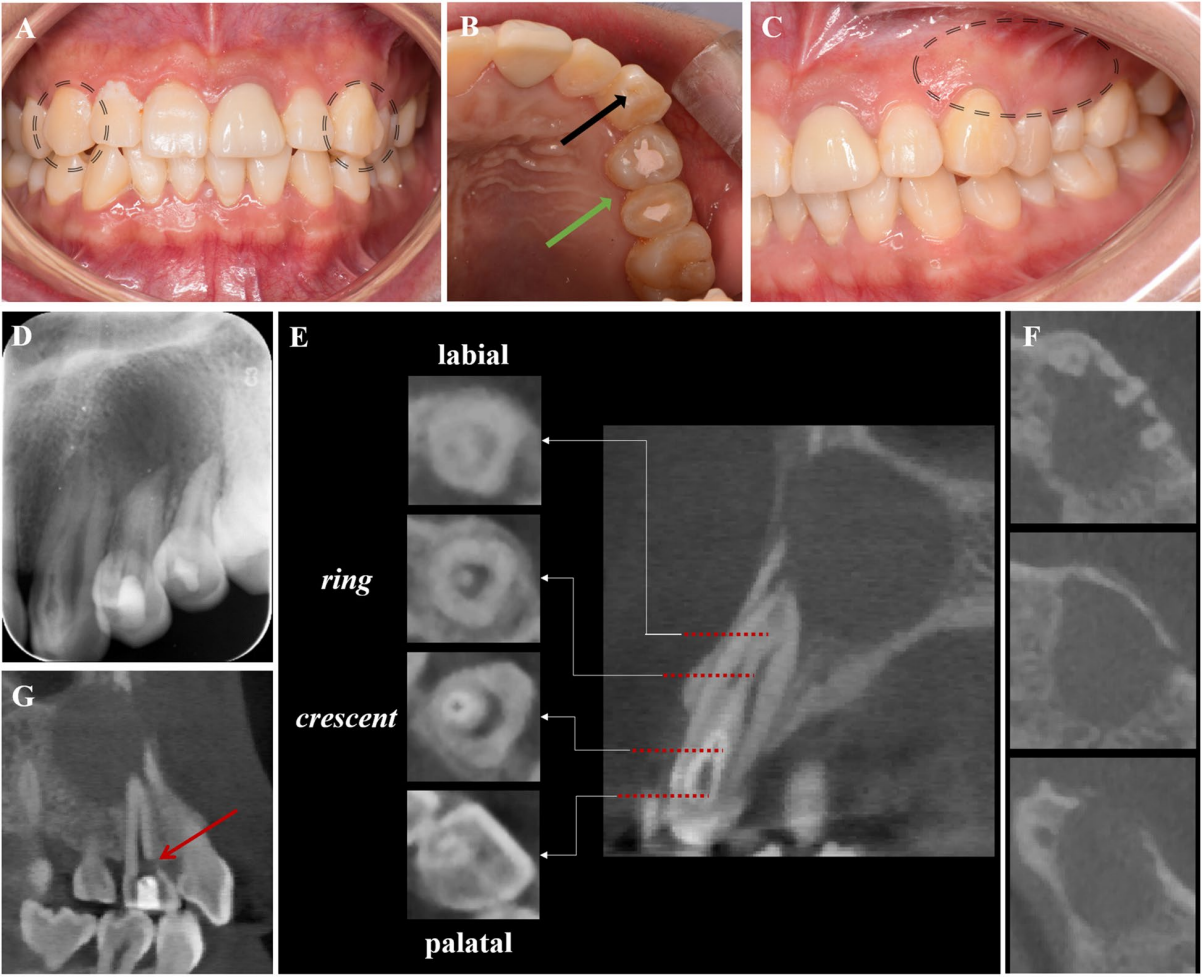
Preoperative examination of case 1. A Tooth 23 exhibited a larger mesiodistal diameter than tooth 13. B Green arrow: temporary sealing material on the occlusal surface of teeth 24 and 25; black arrow: a small foramen caecum on the mesial part of the palatal surface of tooth 23. C No swelling or sinus tract was observed at the buccal/labial alveolar mucosa related to teeth 23-25. D The periapical radiograph revealed CDI in tooth 23 and a radiolucent lesion in the periapical region of teeth 23-25. E-G CBCT scan images: E Axial slices at various points denoted on the sagittal section. The upper half of the invagination appeared tooth-shaped, compressing the true root canal into a crescent, while the lower half showed an irregularly cylindrical shape, compressing the true root canal into a ring. F The periapical radiolucency extended from the mesial side of tooth 23 to the distal side of tooth 25. G A lateral perforation on the mesial wall of tooth 24
